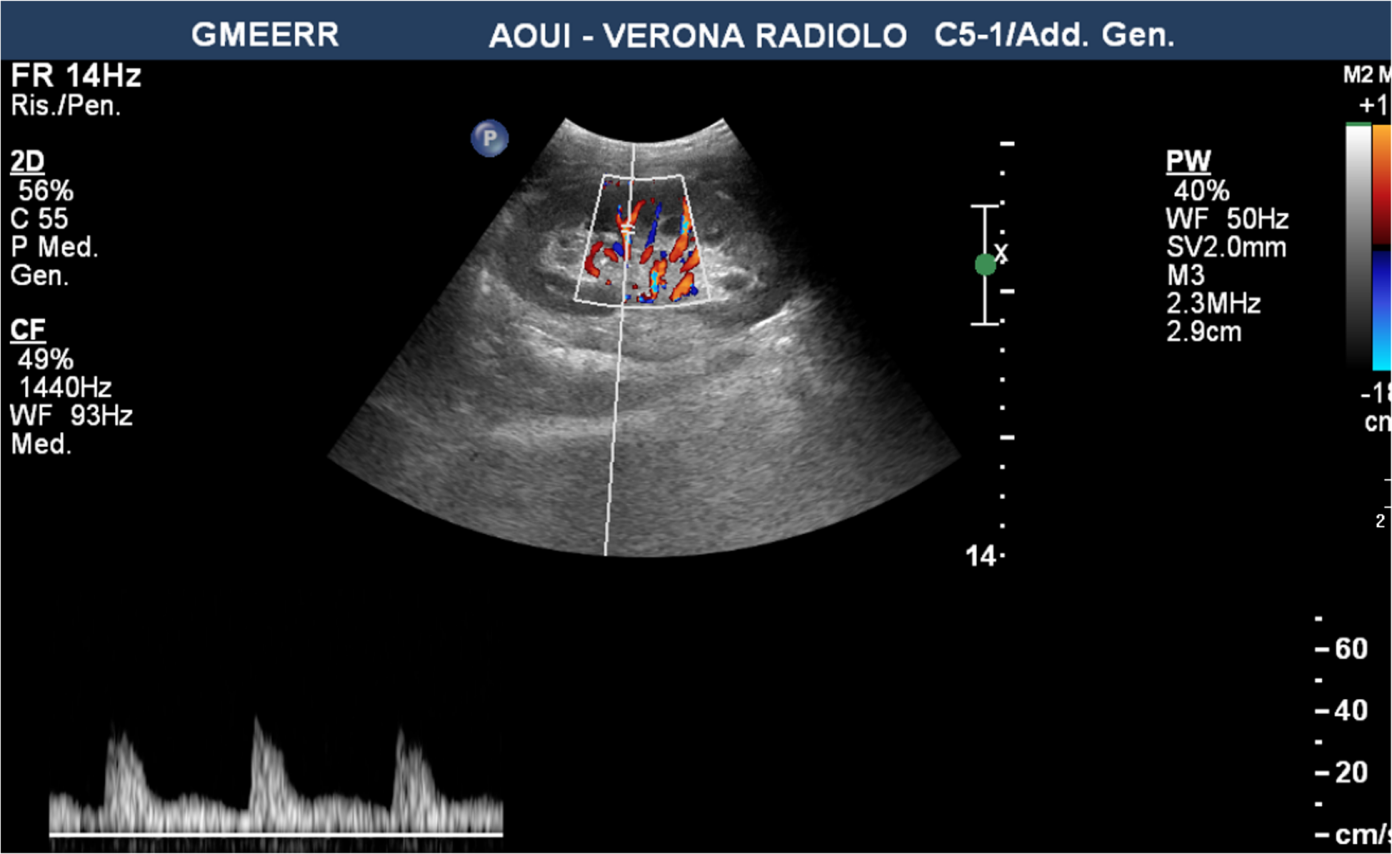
Doppler Ultrasound performed on third postoperative day demonstrating good patency of the vascular anastomoses
